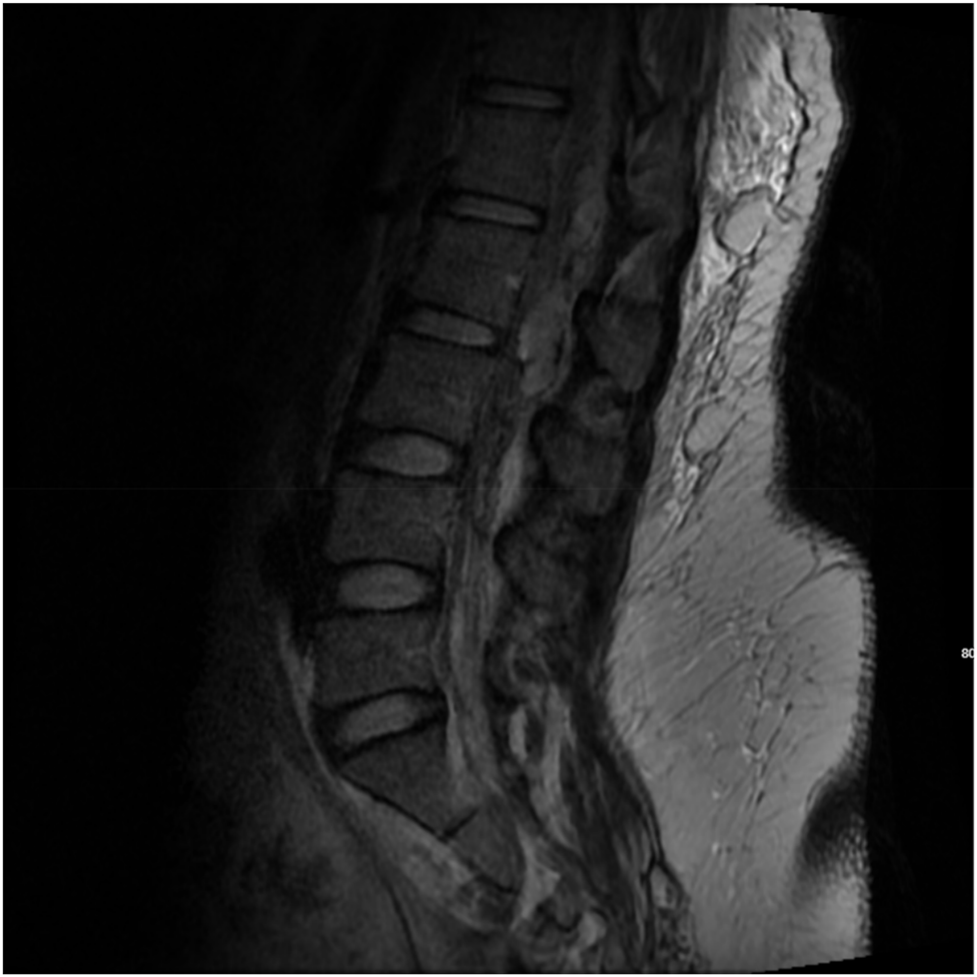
Sagittal MRI of the thoracic and lumbar spine on postoperative day 0. The image reveals a posterior epidural hematoma extending from T12/L1–L4, measuring 1.0 cm anteroposteriorly, 1.1 cm transversely, and 11.5 cm craniocaudally. Significant mass effect on the cauda equina and conus medullaris is evident.
